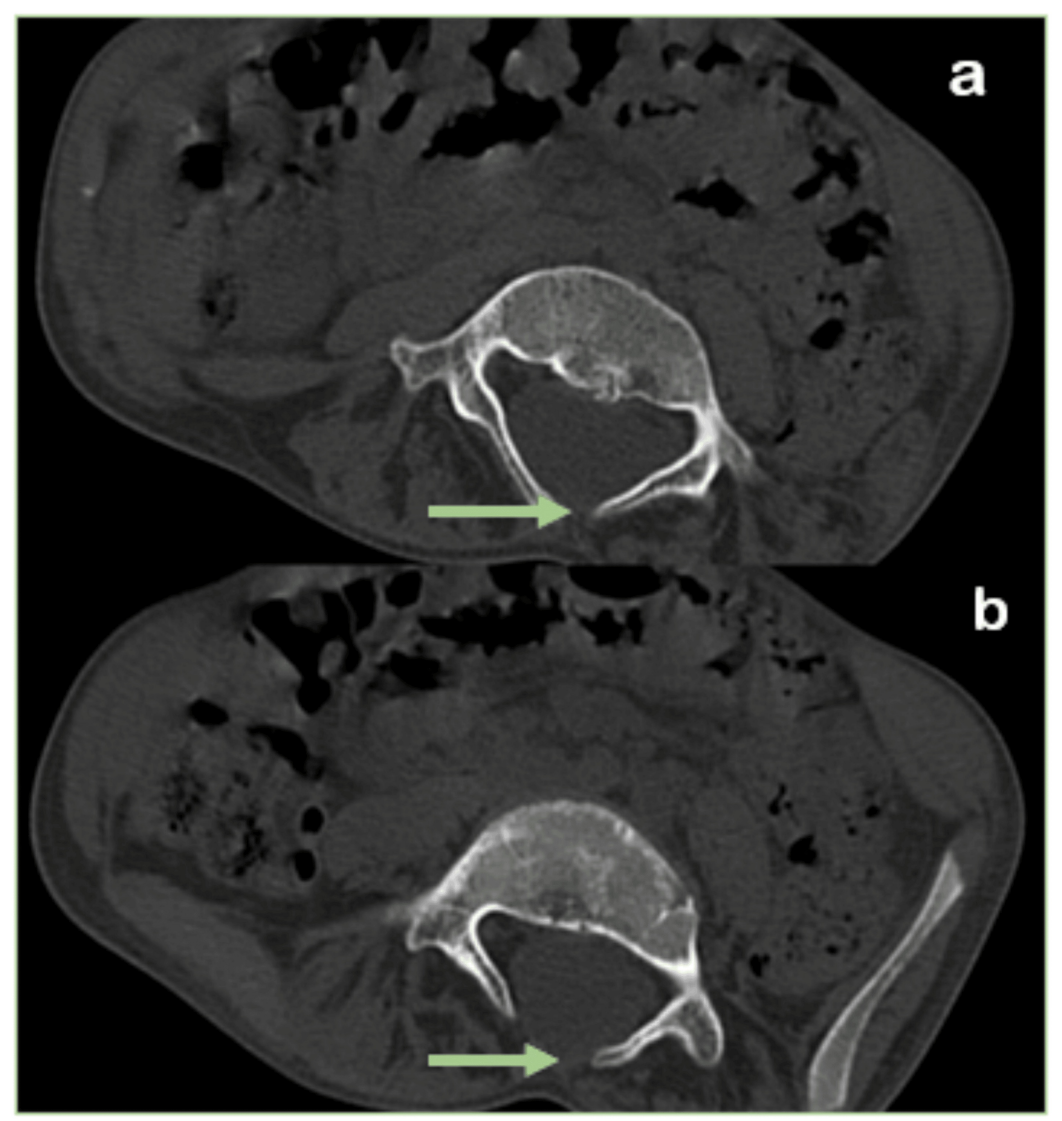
Computed tomography axial section images (a,b) of the spine reveal an unfused spinous process of lumbar vertebrae (green arrow).
